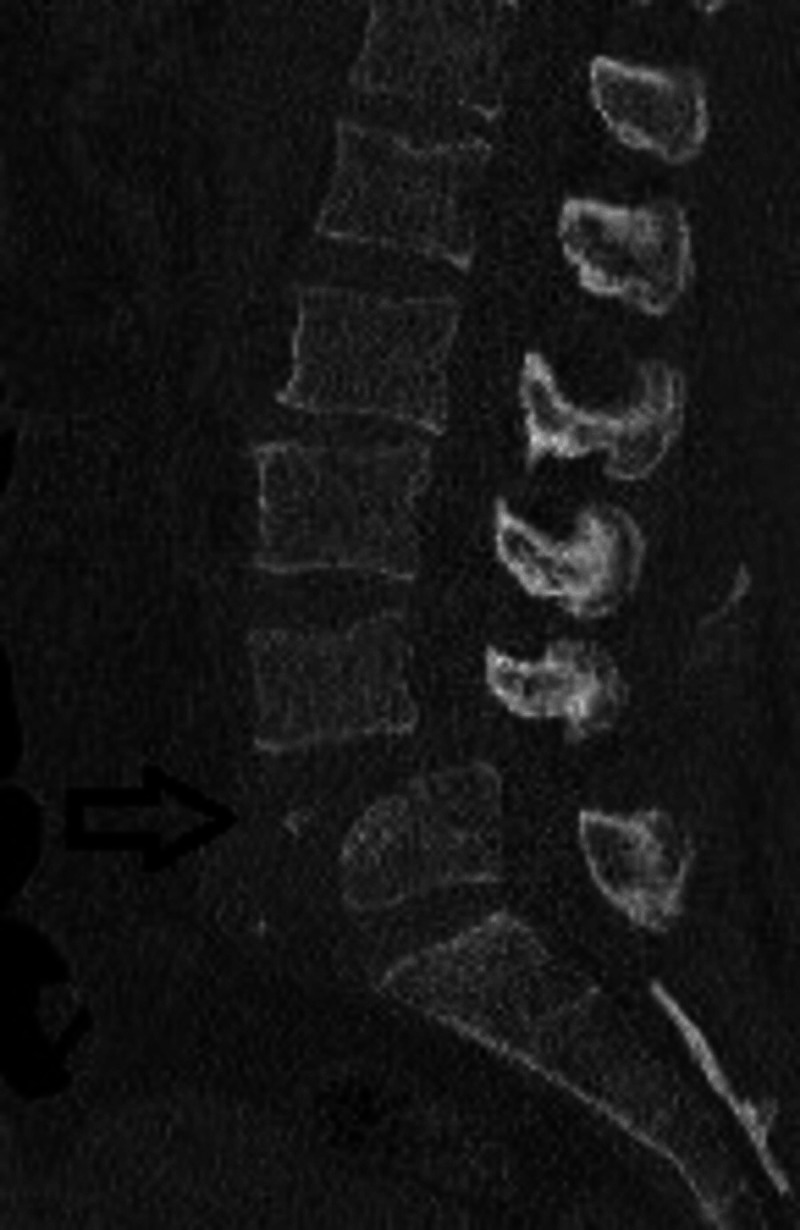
Preoperative lateral supine CT scan with sagittal reconstruction showing the anterolisthesis of L4 and the avulsion L5 fracture (black arrow).
